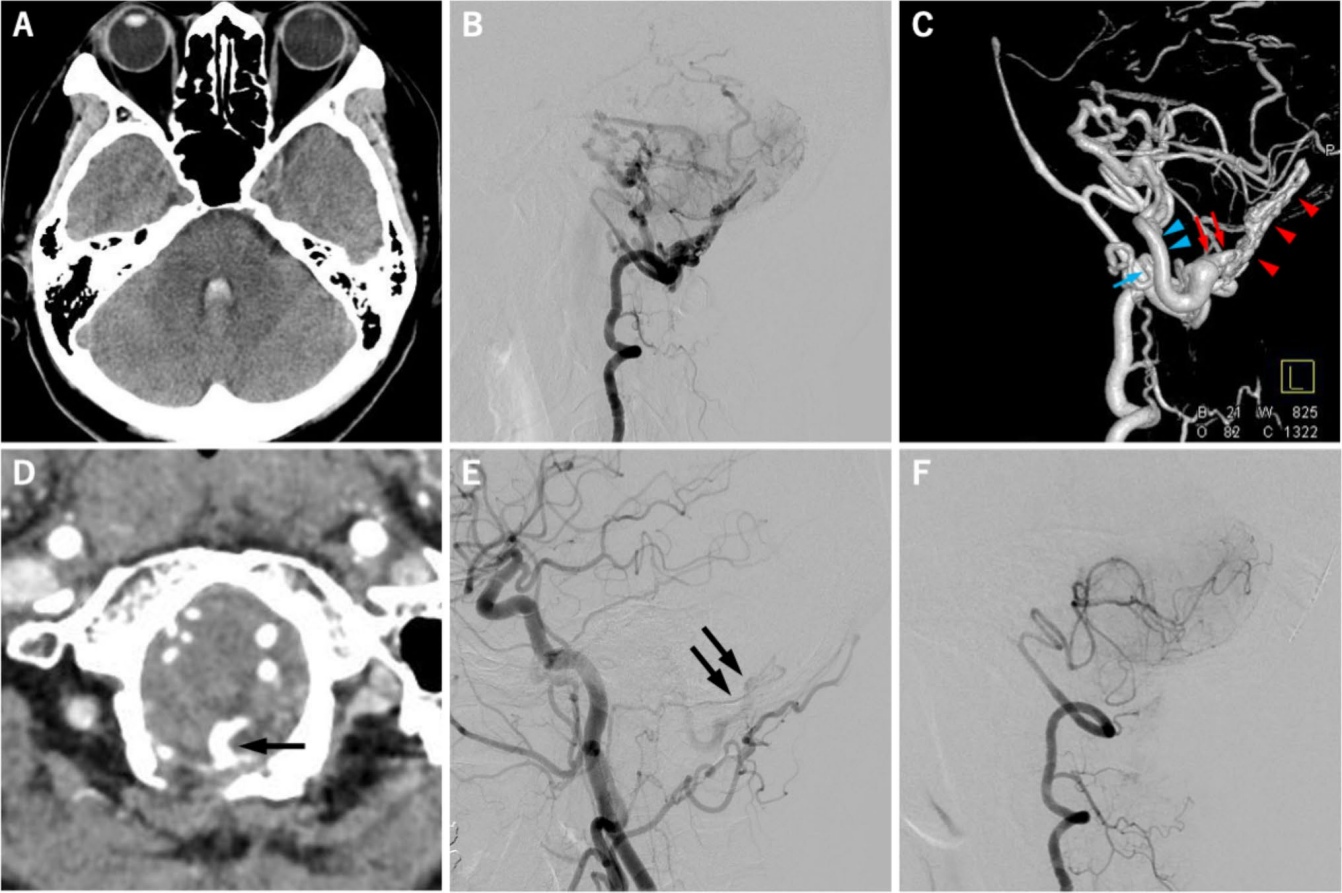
A 60-year-old female presented sudden onset of severe headache. The computed tomography (CT) image on admission showed the small amount of subarachnoid hemorrhage (SAH) and intraventricular hemorrhage (IVH) in the fourth ventricle (a). The lateral view of right vertebral angiogram (VAG) revealed high-flow dural arteriovenous fistula (dAVF) at foramen magnum region (FMR) with marked dilatation of posterior fossa veins (b). Left lateral view of 3-dementional rotational right VAG demonstrated shunted pouch on the surface of dura matter (red arrowhead) and the bridging vein (BV: red arrow) that works as a single drainer of dAVF and connected to vein of inferior cerebellar peduncle (blue arrowhead) and to posterior medial medullary vein (blue arrow) (c). The BV (arrow) that connecting FMR and posterior fossa veins was demonstrated by the source image of CT angiography (d). The lateral view of left carotid angiogram after endovascular transarterial embolization (TAE) of main feeders showed a small amount of residual shunt flow from the dural branches of ascending pharyngeal artery (e). The lateral view of right VAG obtained two weeks after surgical interruption showed the complete disappearance of high-flow FMR-dAVF (f)
